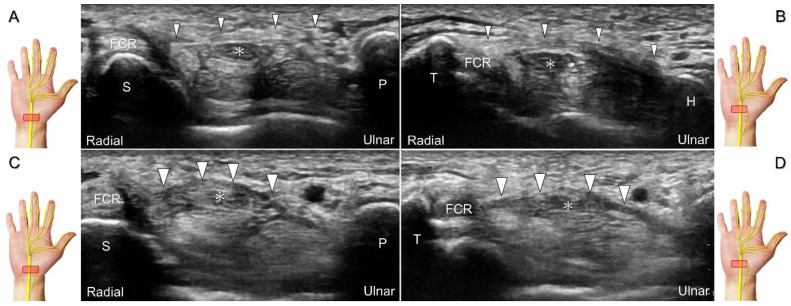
Sonographic/normal imaging of the median nerve from the inlet (A) to the outlet (B) of the carpal tunnel. Hypertrophy of the flexor retinaculum at both the inlet (C) and outlet (D) of the carpal tunnel. Asterisk: median nerve; small white arrowheads: normal flexor retinaculum; large arrowheads: hypertrophy of the flexor retinaculum. FCR: flexor carpi radialis; S: scaphoid; P: pisiform; T: trapezium; H: hook of the hamate.
